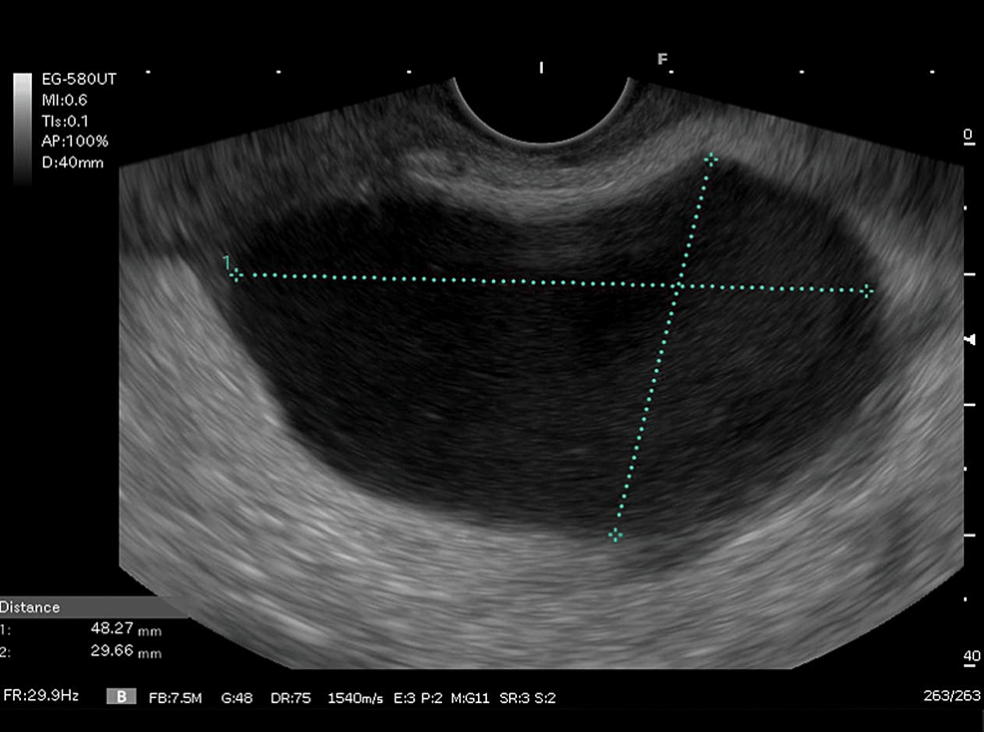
Endoscopic ultrasound showing a 48X30 mm collection that was initially suspected to be a pancreatic pseudocyst but later revealed to be a splenic artery pseudoaneurysm
